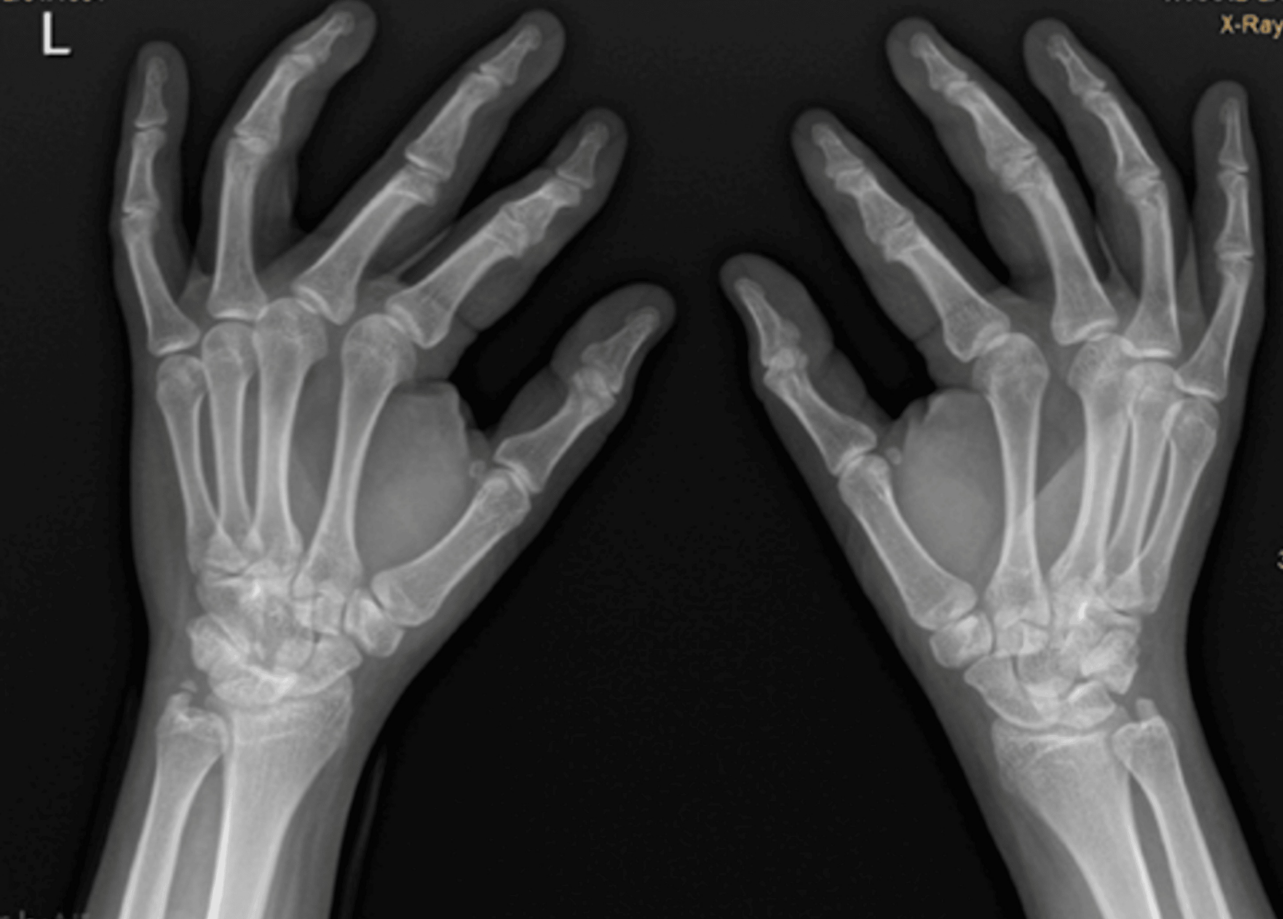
X-ray of the left hand in lateral view.
